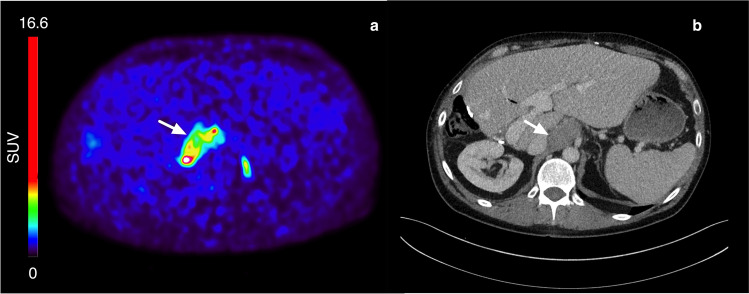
ACC metastasis(arrow), patient 11. [^18^F]CETO PET 3 hours post injection (a) and venous phase contrast-enhanced CT performed 17 days before [^18^F]CETO PET (b). A high uptake of [^18^F]CETO is seen in the ACC metastasis (a)
